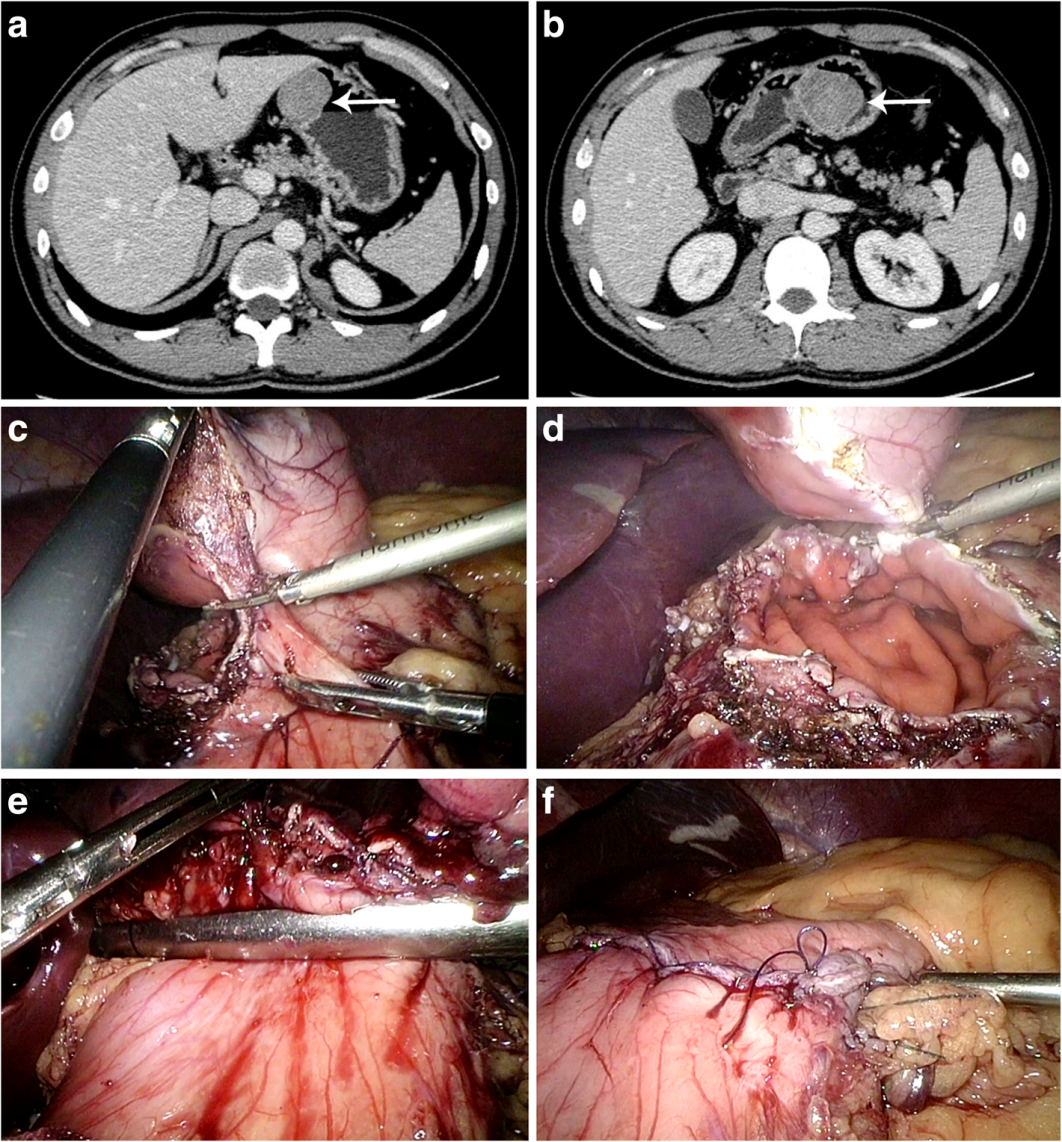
Resection of tumor using ultrasonic coagulating shears. (a) Image of the tumor from abdominal CT scan. (*white arrow*). (b) Image of the tumor from abdominal CT scan. (*white arrow*). (c) Resect along the tumor using ultrasonic coagulating shears. (d) Resect the tumor. (e) Close the opening using endoscopic linear staplers. (f) Reinforce the resection using several sutures
